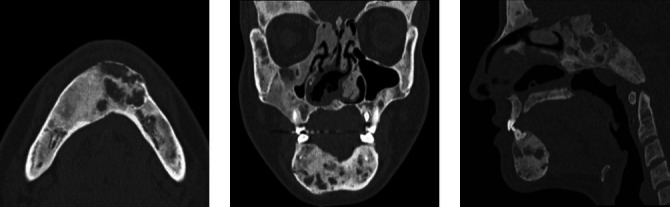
CT scans of a 20-year-old female patient, showing marked bone
